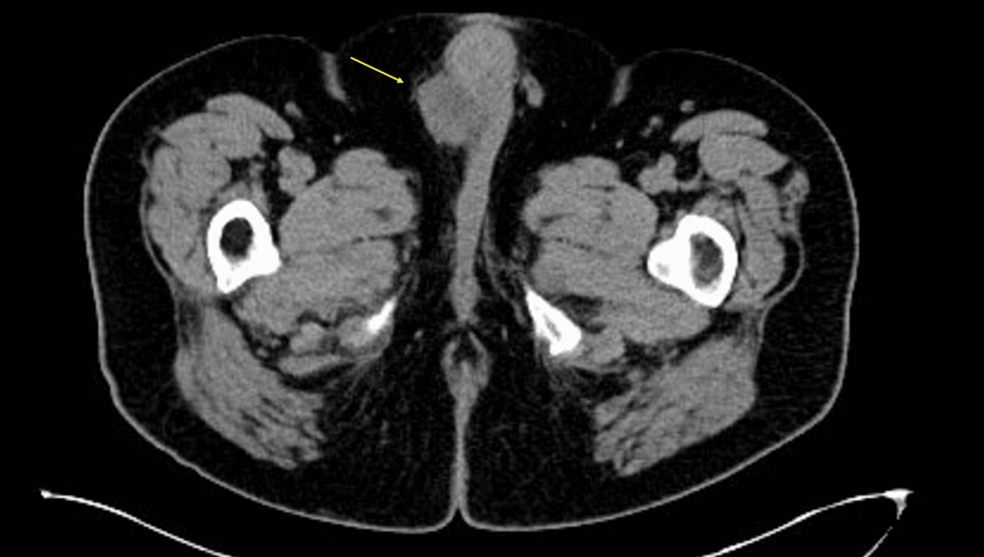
CT of the abdomen and pelvis demonstrating incidentally found partially visualized scrotal lesion (arrow) CT: computed tomography
